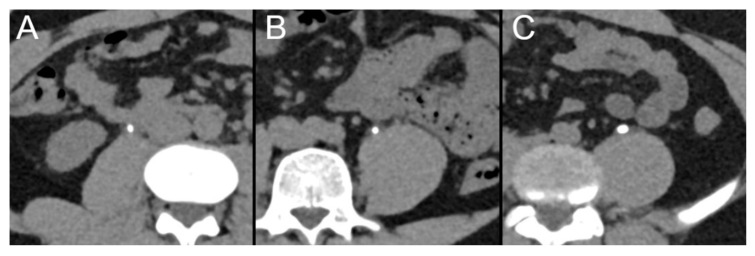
Examples of ureter stones that were included in the analysis of the stone size measurements. CT scans were performed with image quality levels (IQLs) 70 (A), 60 (B) and 50 (C).
